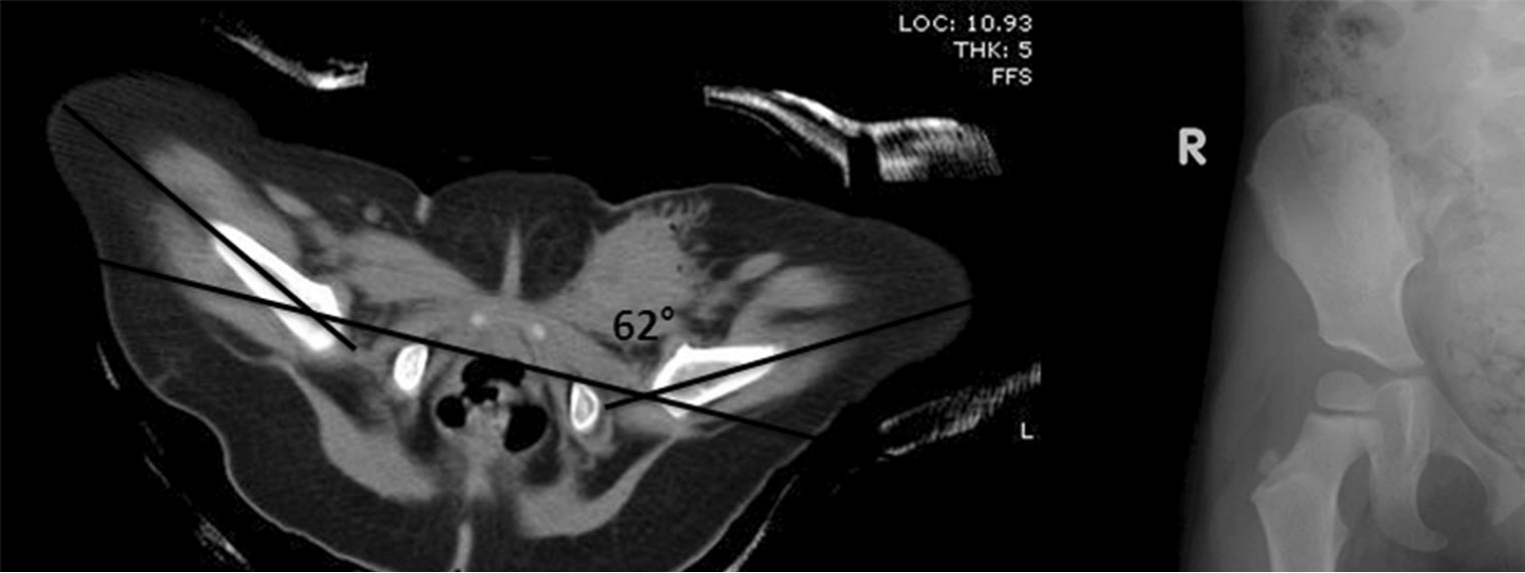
Five-month-old female patient diagnosed with developmental dysplasia of the left hip. The patient underwent failed pre-reduction Pavlik harness treatment for eight days and did not have ossific nuclei present at the time of closed reduction. a Following an adductor tenotomy, closed reduction, and spica cast application, postoperative computed tomography (CT) revealed the left hip to be abducted to 62°. b Radiographs at 1-year follow-up indicate deformity of the left hip indicative of avascular necrosis (AVN). The patient’s final clinical score was graded as fair
